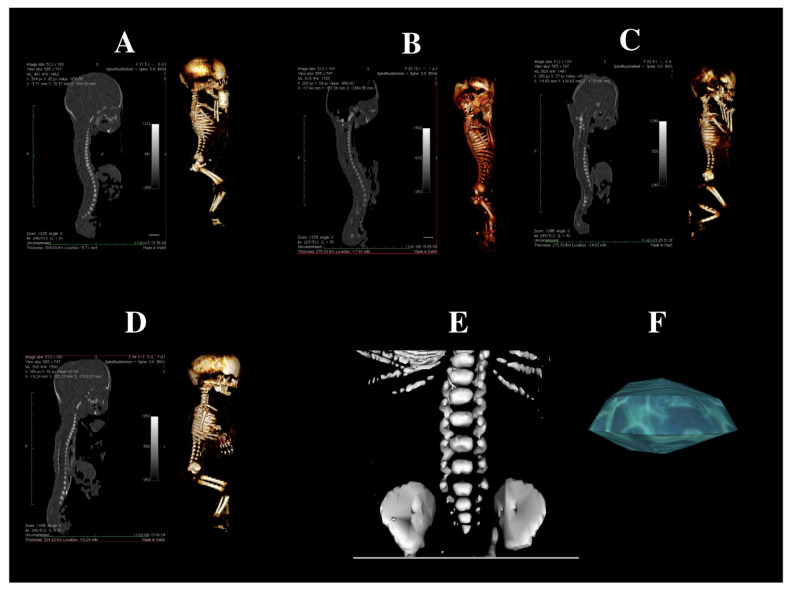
CT scans of a male fetus at 19 weeks (A), 21 weeks (B), 26 weeks (C), and 28 weeks (D) in sagittal projections, as well as 3D reconstruction in sagittal views, are presented. Additionally, a reconstruction of the lumbo–sacral vertebrae of a male fetus at 24 weeks in the frontal projection (E) is shown, including the ossification center of the S1 vertebrae (F) reconstructed using Osirix 3.9.
